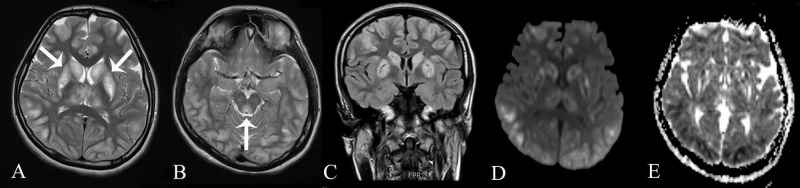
Magnetic Resonance Imaging (A) Axial T2-weighted image showing similar symmetrical T2 hyperintense lesions in cortical and deep grey matter (white arrows). (B) Axial T2-weighted image showing involvement of the periaqueductal grey (white arrow). (C) Coronal FLAIR image showing that the lesions were hyperintense on FLAIR. (D & E) Diffusion-weighted sequences and apparent diffusion coefficient maps showing patchy diffusion restriction in the affected regions.
